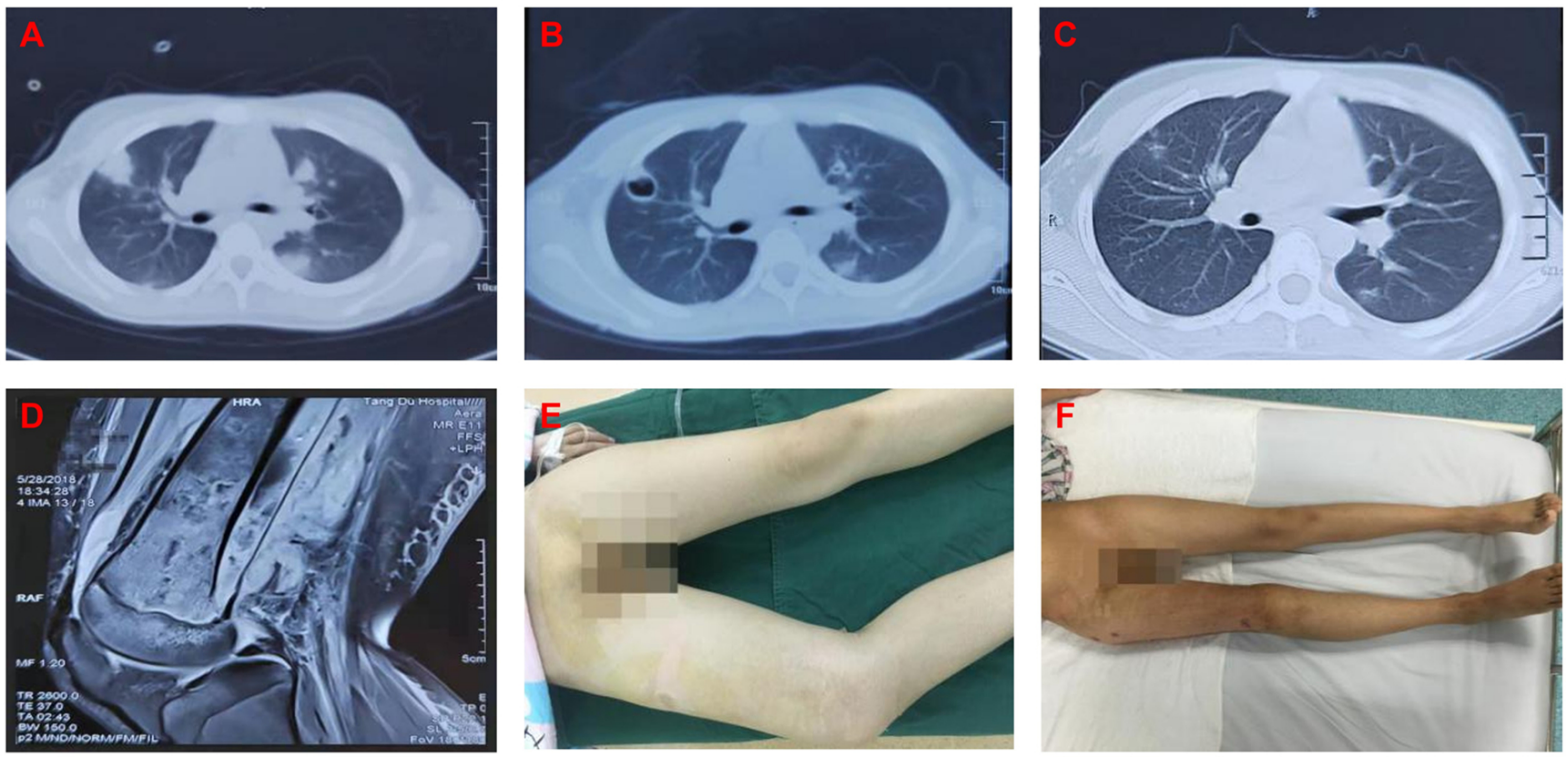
(A) 2018.05.20 lung CT(Before surgery): It was indicated that there were multiple abscesses in the lung. (B) 2018.05.28 lung CT(Before surgery): It was indicated that the lung abscesses shrinked after treatment. (C) 2018.06.20 lung CT(After surgery): It was indicated that the lung abscesses almost disappeared. (D) 2018.05.28 MRI(Before surgery): It was indicated that there were edemas in the medullary cavity of the metaphysis soft tissue and surrounded the distal femur. (E) General photo at admission: It was indicated that forced posture caused by the disease and limb swelling. (F) General photo at discharge: It was indicated that the right leg of the patient was free of pain and swelling, the hip and knee joints could move freely as well.
